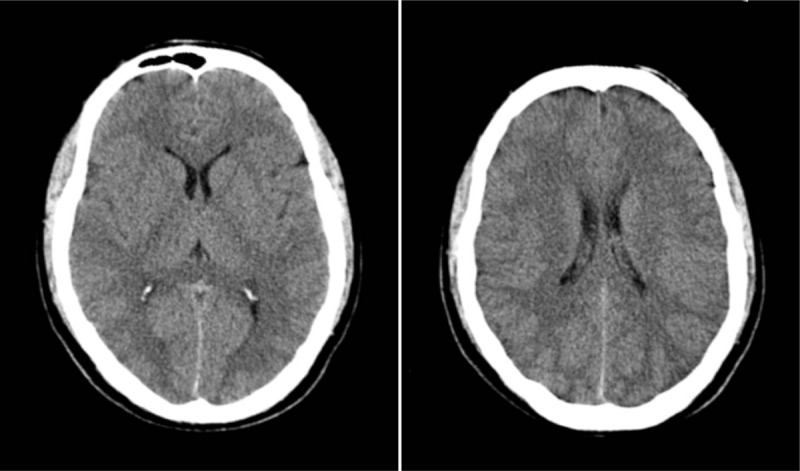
Head computed tomography showing reduction in brain density.
